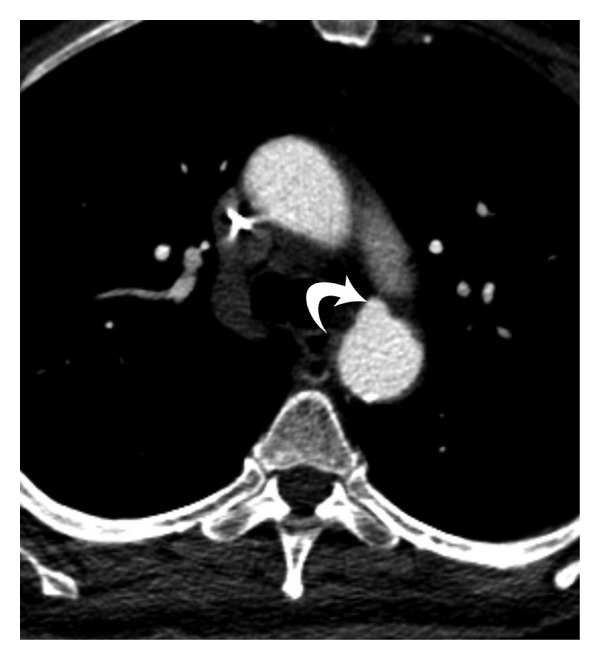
Acute  traumatic  aneurysm—postcontrast axial CT scan image in a 28-year-old male who was involved in a motor vehicle accident shows a small focal outpouching from the anterior aspect of the proximal descending thoracic aorta (curved arrow), which is indicative of aortic trauma. Note the absence of mediastinal hematoma in this patient.
